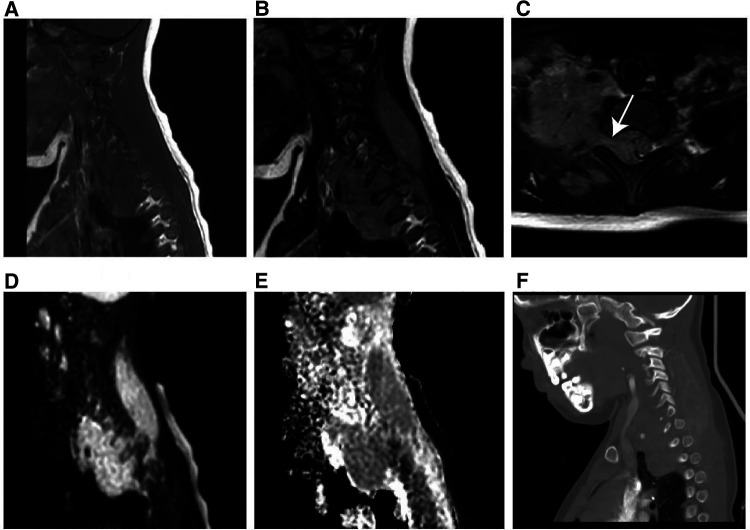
MR imaging results of a 60-month-old girl with RMS (clinical manifestations: unintentionally found a mass in the right neck for 20 days, accompanied by pain in the right upper limb for 15 days). (A) T1-weighted MR imaging revealed an isointense soft tissue mass. (B) T2-weighted MR imaging revealed a hyperintense mass. (C) Axial T2-weighted MR imaging revealed that the mass had expanded into the cone through the intervertebral foramen. (D) Hyperintensity on the DWI image. (E) The mean apparent diffusion coefficient value of the mass was 1.441 × 10^−3^ mm^2^/s. (F) The sagittal CT image revealed no obvious bone destruction.
